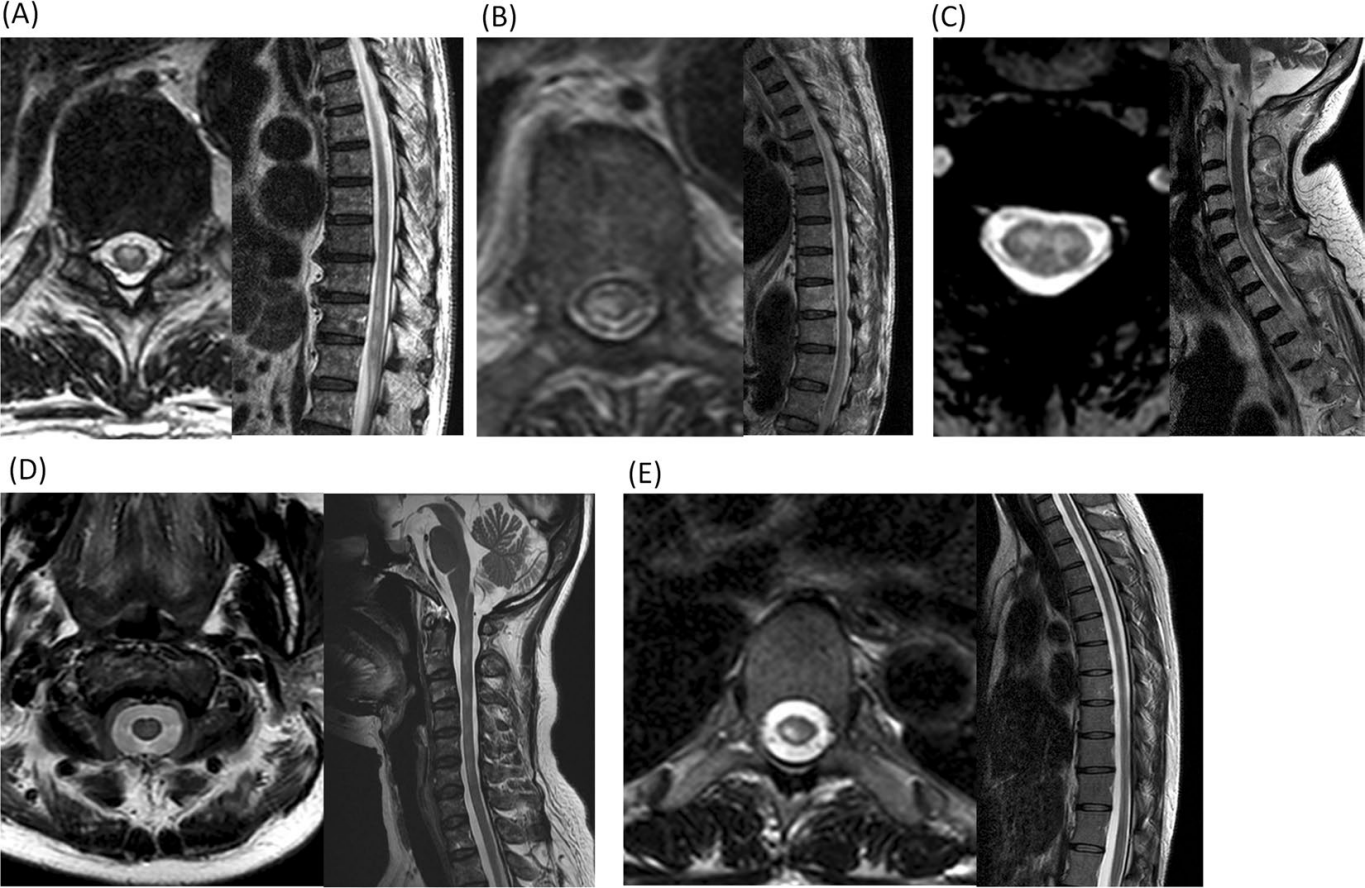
Typical MRI scans of acute myelopathy due to SCI and NMOSD. (A) An 83-year-old man had an infrarenal abdominal aorta aneurysm. After operation, he suffered from acute paraparesis with urine incontinence. His spine MRI scans showed a typical ASA infarction (axial view) at the T10–T12 levels (sagittal view). (B) A 79-year-old man had a history of abdominal aortic aneurysm dissection. After operation, he had acute paraplegia with urine incontinence. His spine MRI scans showed combined ASA and right PSA infarctions (axial view) at the T8–T10 levels (sagittal view). (C) A 57-year-old woman had a sudden onset of quadriparesis with neck pain and urine incontinence. The MRI scans showed a typical owl’s eyes sign (axial view) at the C5–7 levels (sagittal view). (D) A 49-year-old female had progressive paraparesis for 7 days. Her spine MRI scans showed transverse myelopathy (axial view) at the C2–C6 levels (sagittal view). (E) A 45-year-old female had a subacute onset of progressive paraparesis. Her MRI scans showed typical bright spotty lesions (axial view) and a hyperintense lesion at the T2–T4 levels (sagittal view).
